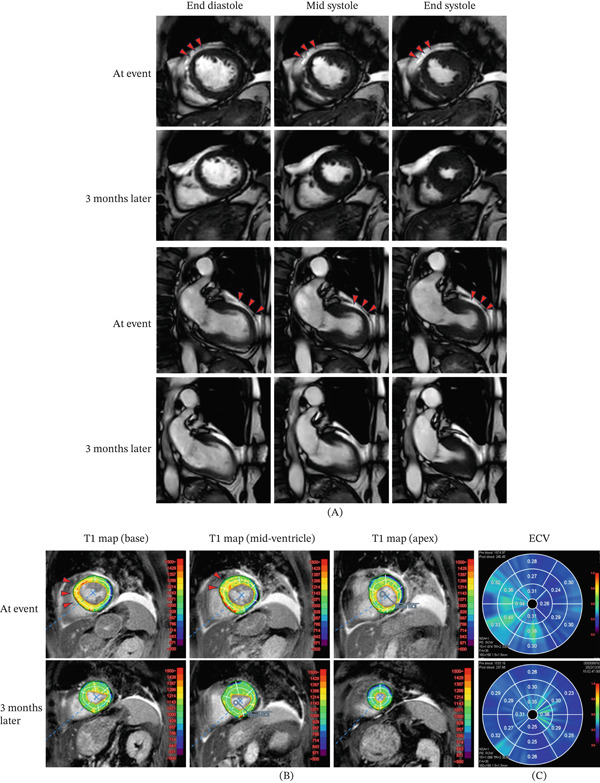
Cardiac magnetic resonance images with time course. (A) Cine MRI with time course. The red arrowheads indicate the area of severe hypokinesis in the left ventricle. (B) T1 mapping at the event and 3 months later. The red arrowheads show the area with high native T1. (C) Bull′s eye images of extracellular volume fraction at the event and then at 3 months later.
